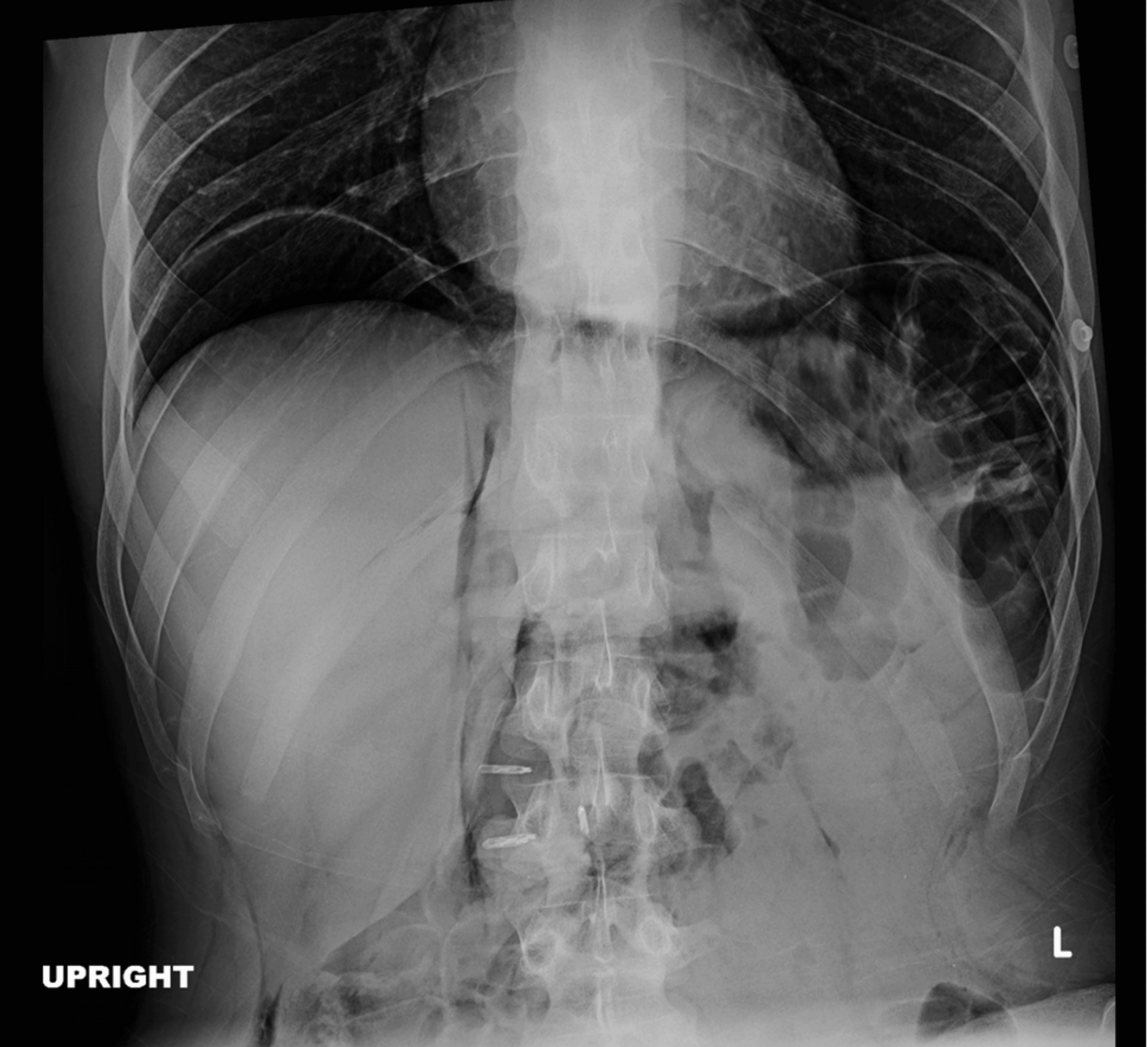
X-ray with diffuse pneumoperitoneum Upright abdominal X-ray obtained after esophageal and gastric insufflation during endoscopy showed diffuse pneumoperitoneum.
